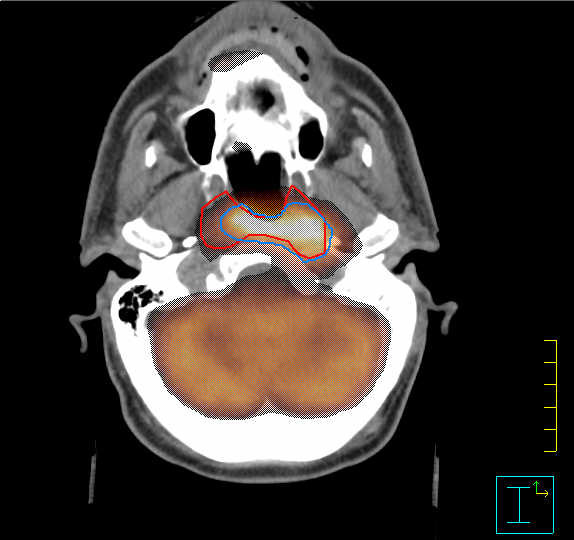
Axial FDG-PET/CT image of a patient with nasopharyngeal undifferentiated carcinoma. The computed tomography gross tumor volume (CT-GTV) and the positron emission tomography (PET)-GTV are highlighted with red and light blue contours, respectively. For treatment purposes both findings were taken into account.
